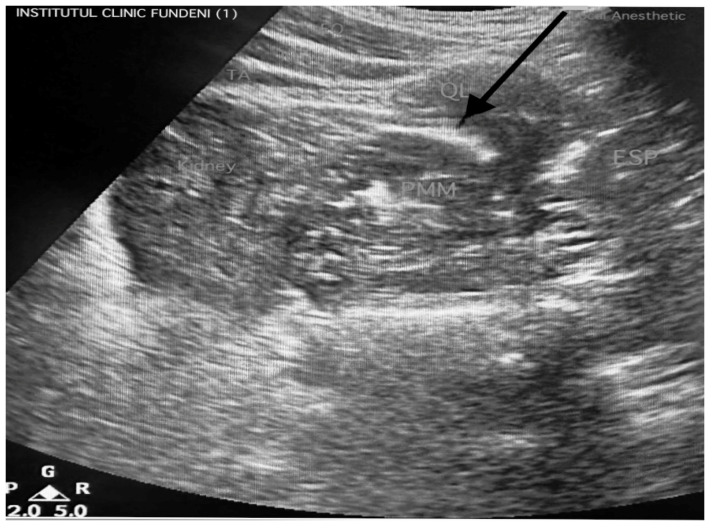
Ultrasound QL block approach: the needle is advanced in an anterolateral-to-posteromedial direction under US guidance to ensure precise localization. Bilateral type 3 (anterior) QL blocks were administered, with the injectate deposited at the fascial plane between the anterior border of the QLM and the PMM (from the authors’ collection). The arrow indicates the direction and the site of injection for the local anesthetic.
